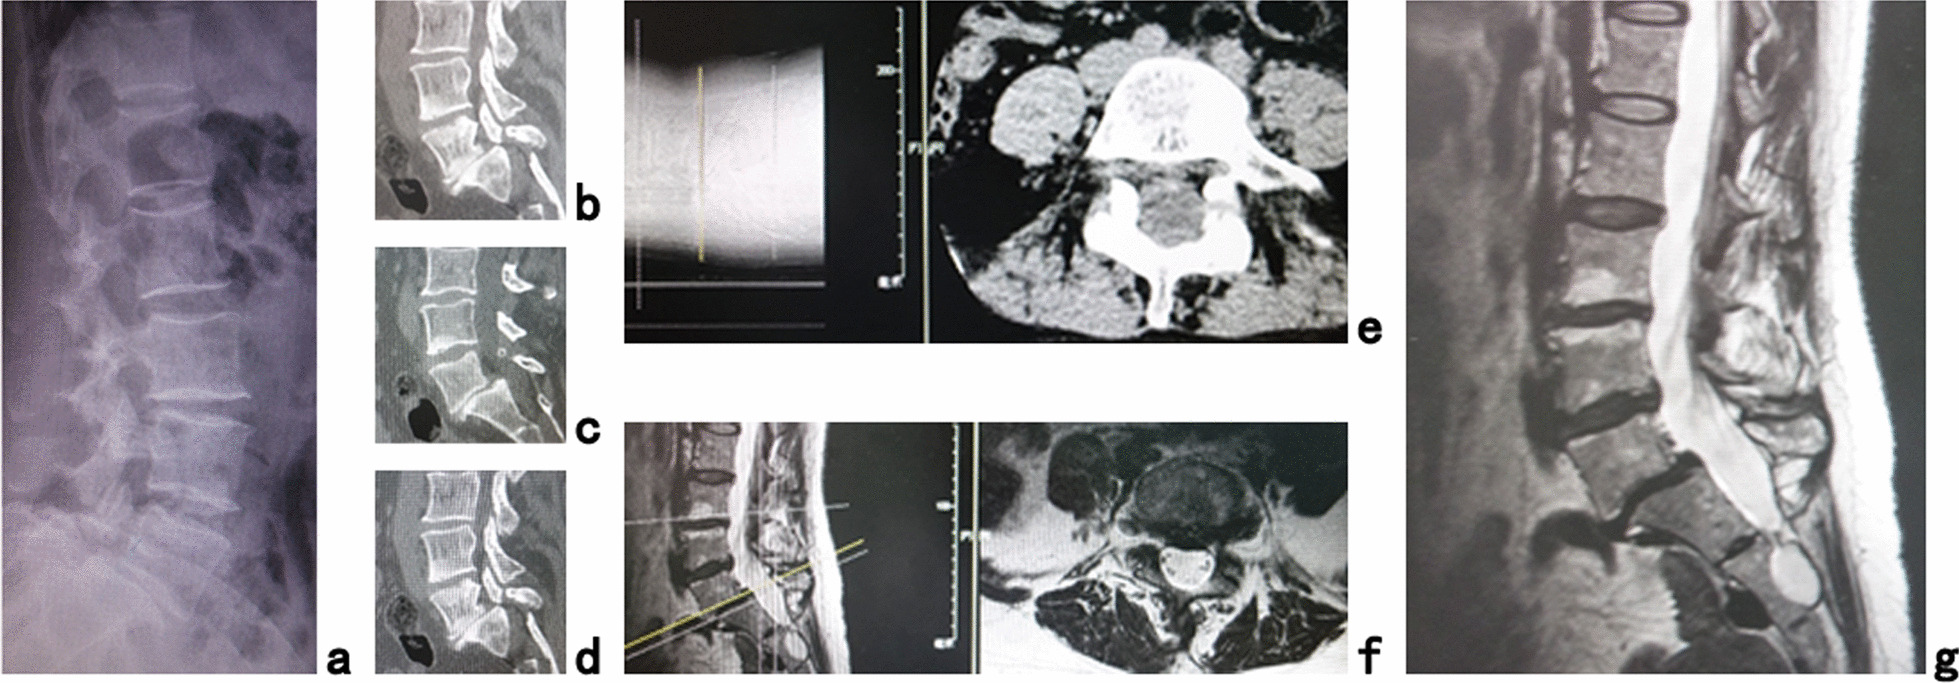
A 35-year-old male patient had low back pain and right leg pain for 1 year. a Lateral X-ray, b–d sagittal CT, e axial CT, f axial MRI and g sagittal MR images showed L5 spondylolisthesis (Meyerding II°) with bilateral spondylolysis and lateral recess stenosis at L5/S1 (Case 3)
